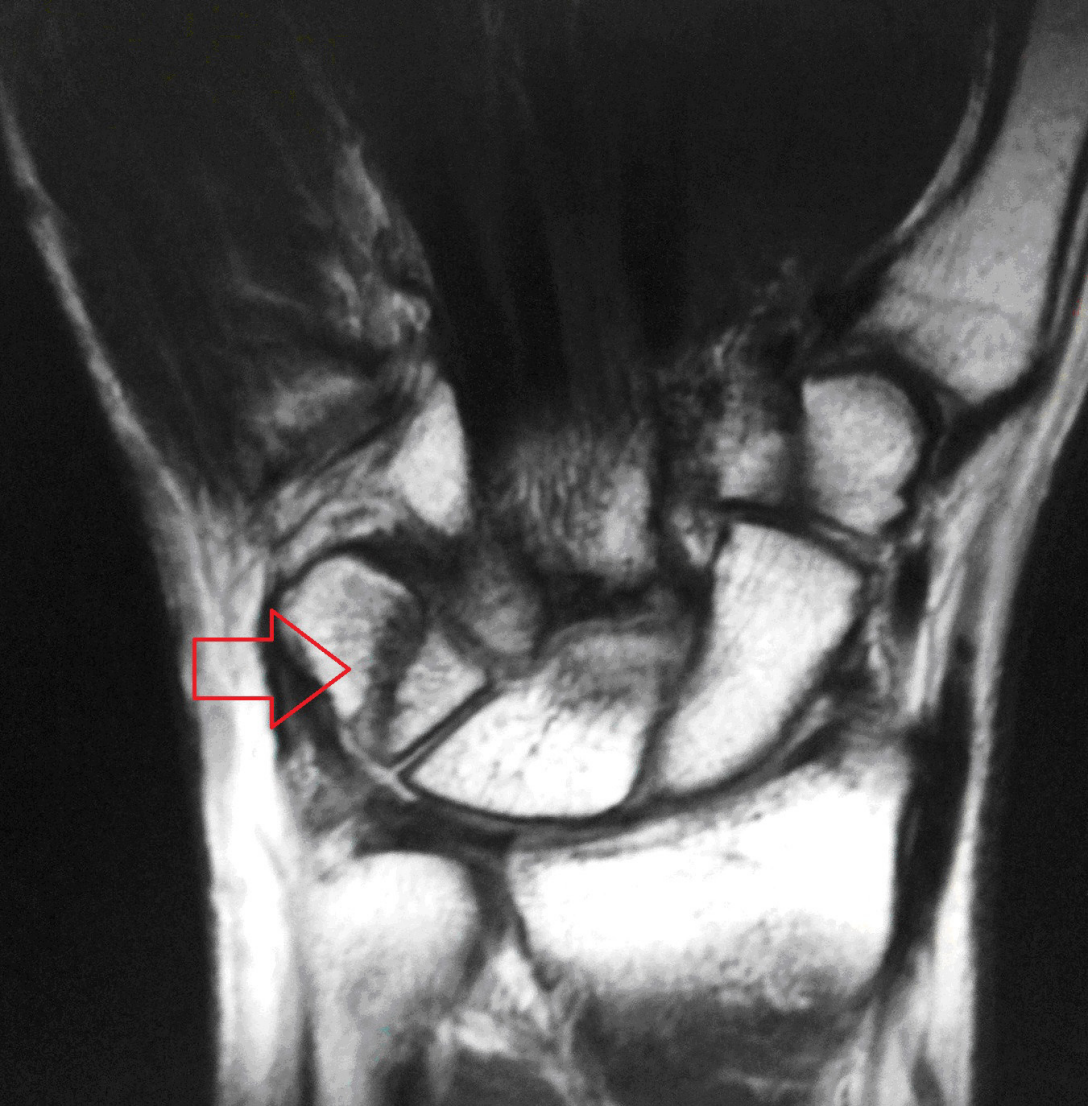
Coronal magnetic resonance imaging scan of the right wrist at five months after the injury. The image shows a non-united, displaced, and isolated fracture of the body of the triquetrum.
